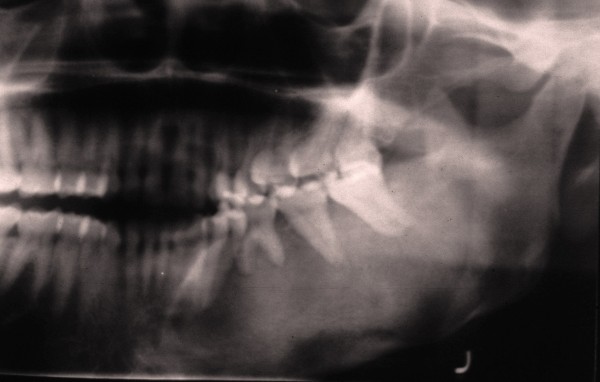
Cropped panoramic radiograph of fibrous dysplasia of the left mandible. Note the diffuse mottled-glass appearance and tooth displacement.
